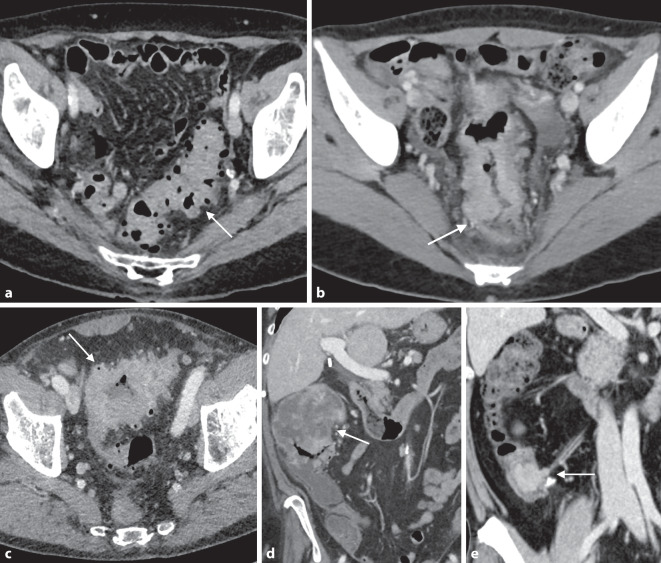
Die Rolle der präoperativen Bildgebung zur Beurteilung des lokalen Tumorstadiums wird noch untersucht, da die neoadjuvante Behandlung und Strahlentherapie die Überlebensrate im Vergleich zur operativen Resektion bisher nicht signifikant verbessert haben. Eine sichere Differenzierung zwischen einer rein mukosal/submukosalen Läsion (T1) und einer die Muscularis propria infiltrierenden Läsion (T2) ist derzeit bildgebend nicht möglich. Eine Infiltration der perikolischen Strukturen (T3) ist durch eine Verdichtung des angrenzenden Fettgewebes und eine unscharfe Begrenzung der Außenwand des Dickdarms mit zum Teil nodulären Tumorausläufern in das perikolische Fettgewebe erkennbar. Die CT weist in einer Metaanalyse eine gepoolte Sensitivität von 90 % für die Detektion einer Wandüberschreitung auf, mit einer Sensitivität von 77 % für die Abgrenzung einer perikolischen Invasionstiefe > 5 mm, entsprechend einem T‑Stadium > T3b. Die CT-Kolonographie zeigt dabei eine höhere Genauigkeit in der Differenzierung der Tumorstadien T1–T3ab von T3cd–T4 [13]. Aufgrund der begrenzten Rolle des lokoregionären Stagings liegt der primäre Fokus der präoperativen Bildgebung des Kolonkarzinoms daher auf der Beurteilung der Umgebungsinfiltration und dem Vorhandensein von Fernmetastasen [14].
